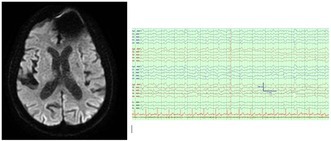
FIGURE 1 MRI and EEG of the first pation explained.
